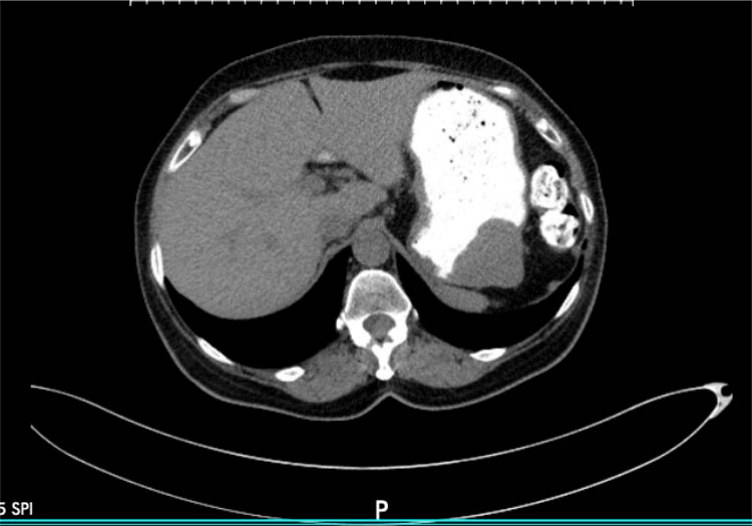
Axial view of the abdominopelvic CT scan with oral contrast showing a well-circumscribed gastric soft tissue attenuation. P: Posterior.
